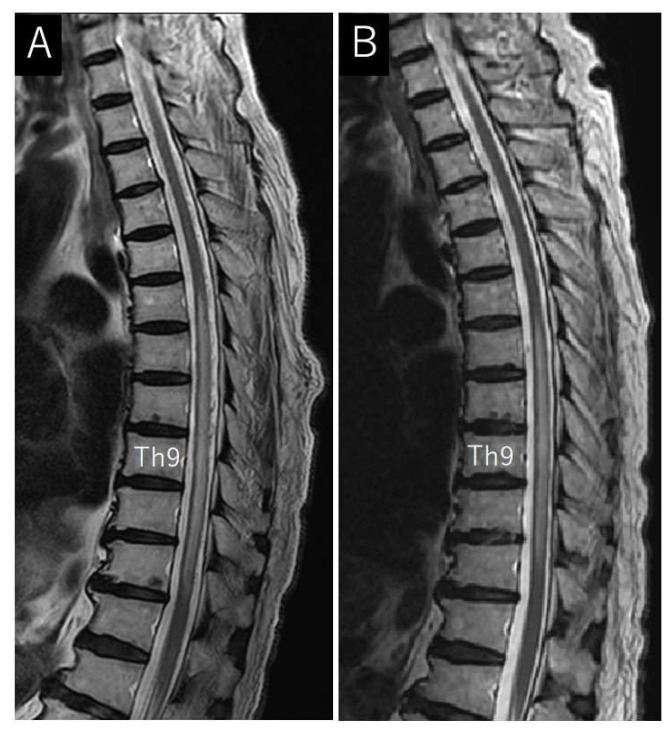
Pre- and post-treatment magnetic resonance (MR) images from a patient with dural arteriovenous fistula (DAVF); (A) pretreatment sagittal T2-weighted MR image of the thoracolumbar spine showing spinal cord edema and flow voids around the cord; (B) posttreatment sagittal T2-weighted MR image of the thoracolumbar spine showing edema reduction and flow-void disappearance.
